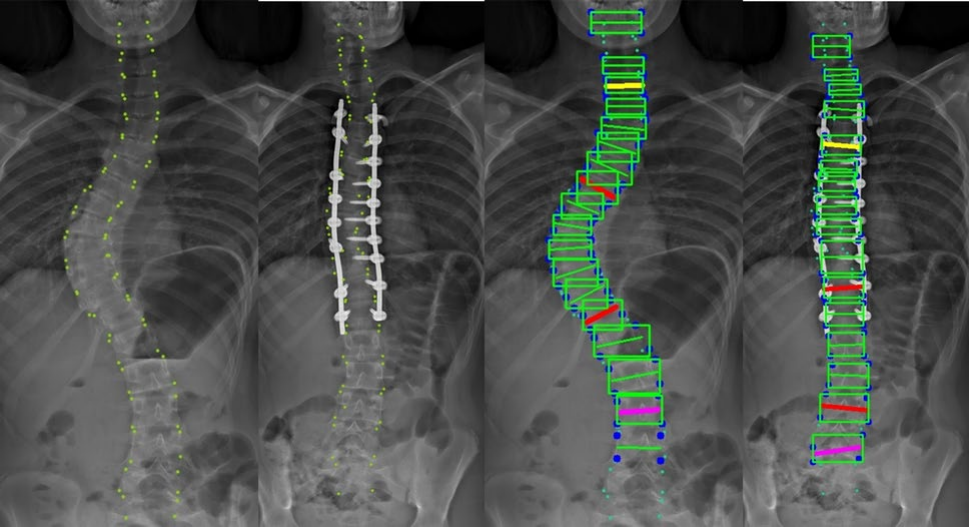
Whole-spine standing radiographs of a patient undergoing long-segment posterior spinal fusion for adolescent idiopathic scoliosis correction. The images display preoperative coronal annotations (A), postoperative coronal annotations (B), preoperative coronal predictions (C), and postoperative coronal predictions (D), including keypoints and bounding boxes
